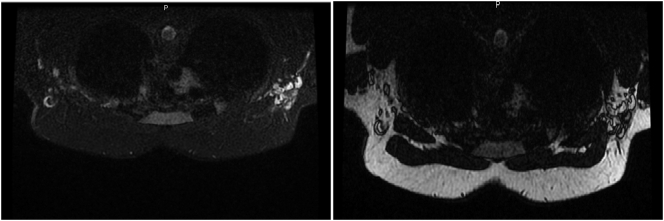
Breast MRI after neoadjuvant chemotherapy, maintaining right axillary adenopathic conglomerate, without breast lesions.
